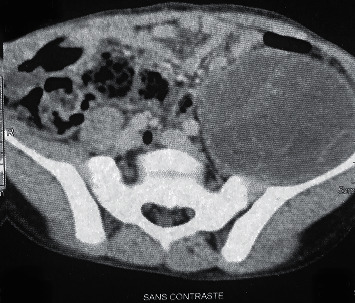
CT scan showing a well-limited hydatid cyst in the expansion of left psoas muscle.
